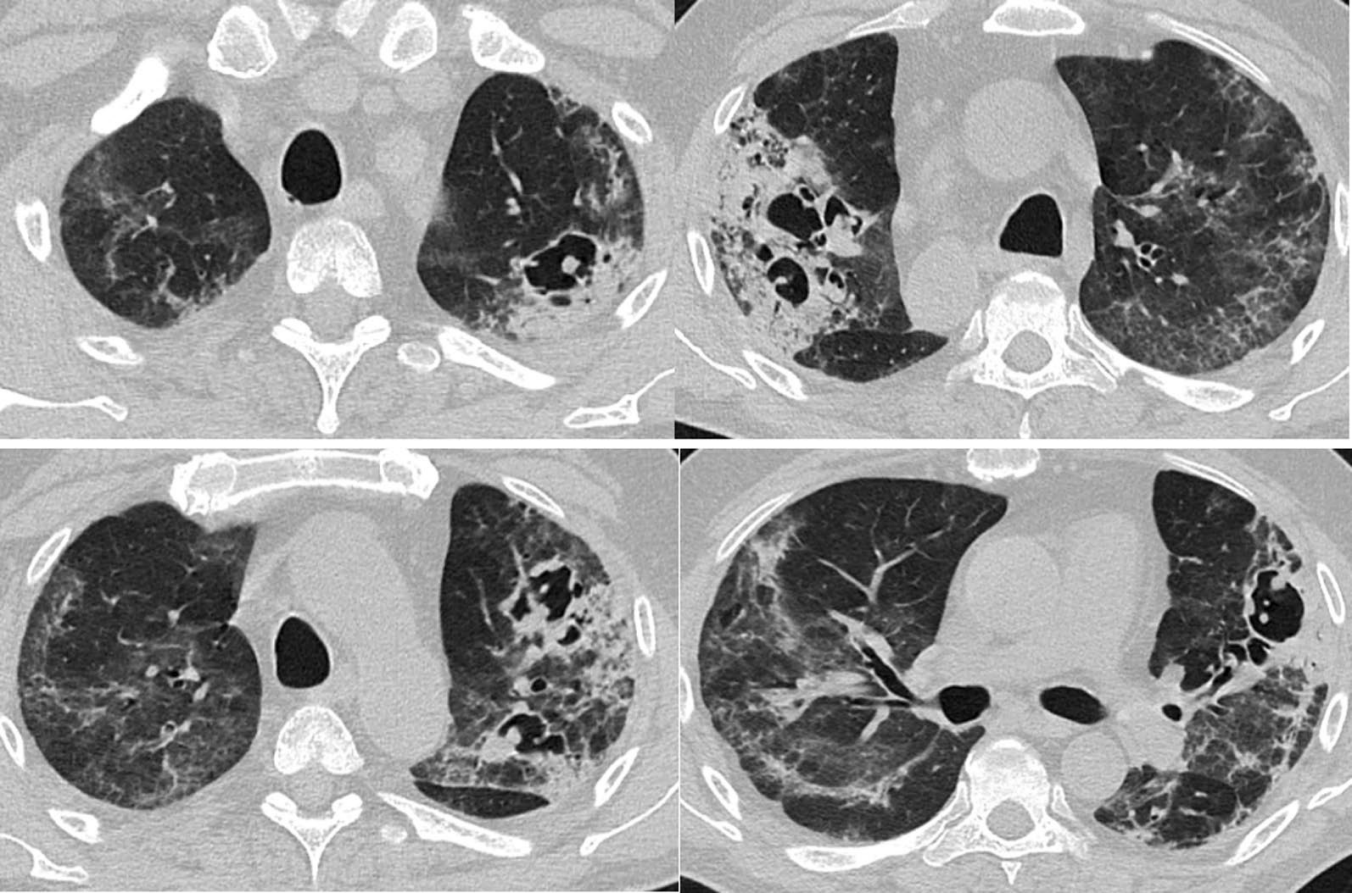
Axial HRCT images of case 6 shows multiple cavitary lesions in both lungs with irregularly margined soft tissue attenuation nodular growth. Sputum culture revealed Candida species
